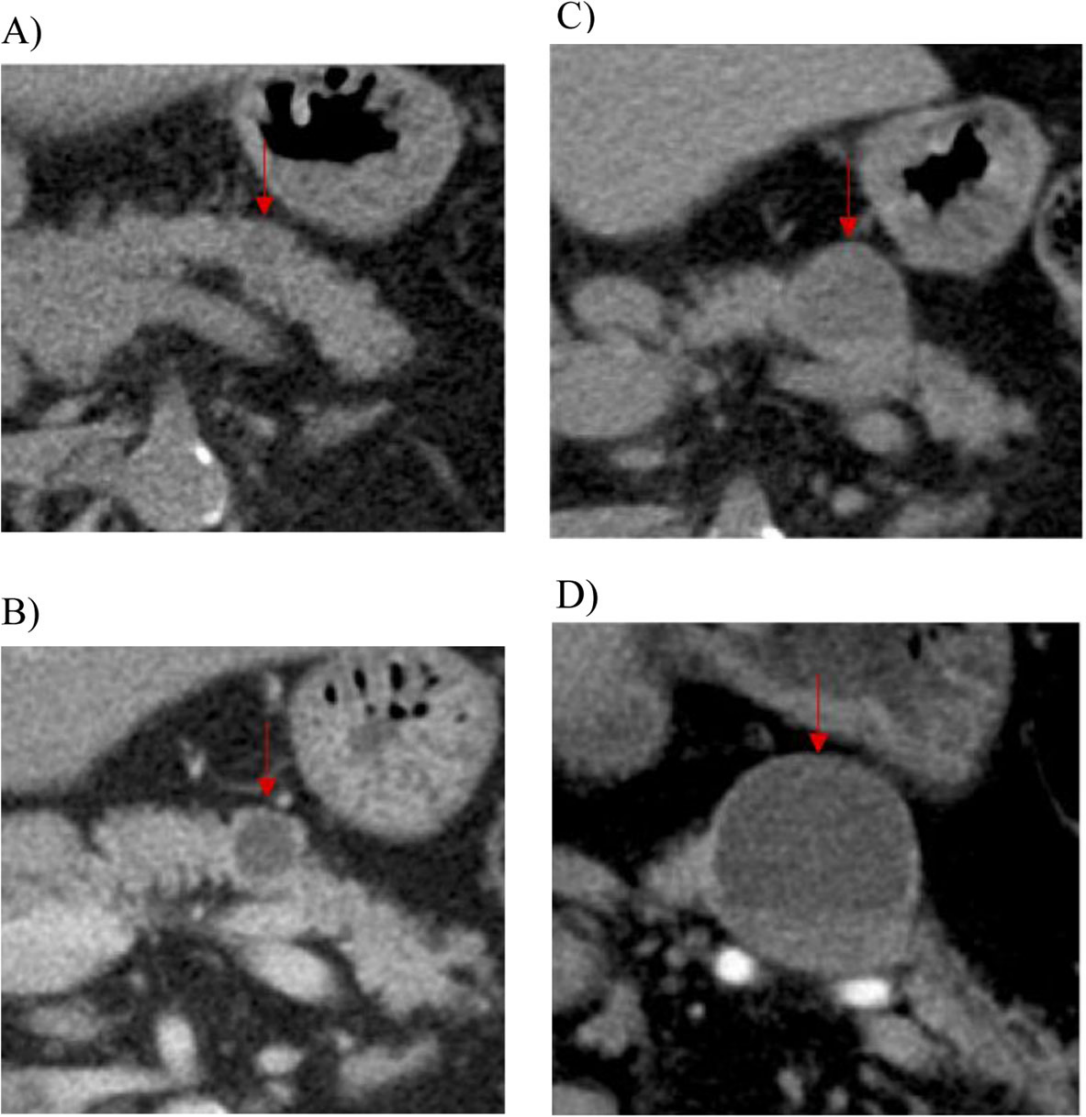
Abdominal CT showing the cystic lesion in the tail of the pancreas that had become larger without a change in form. a Unenhanced CT showing a 9-mm cystic lesion in 2011. b Contrast-enhanced CT (CECT) showing a 14-mm cystic lesion in 2013. The peripheral rim was enhanced. c Unenhanced CT of a 33-mm cystic lesion and consisting of fluid at the bottom, which was considered clot in 2018. d CECT showing a 40-mm cystic lesion in 2019. The outer rim became thinner
